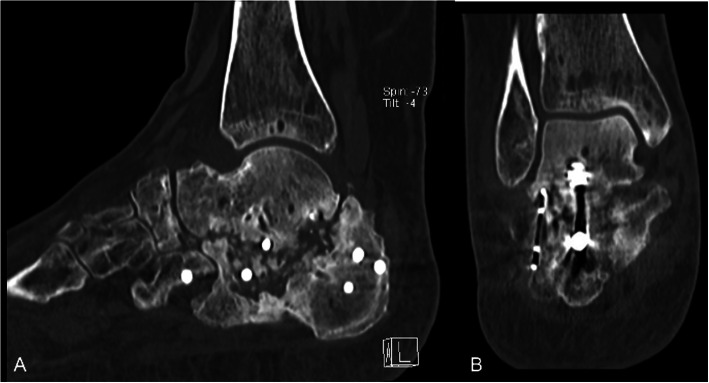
Case 2 Complications. CT scan sagittal (A) and coronal (B) view 6 months after primary subtalar fusion showing a subtalar non-union
